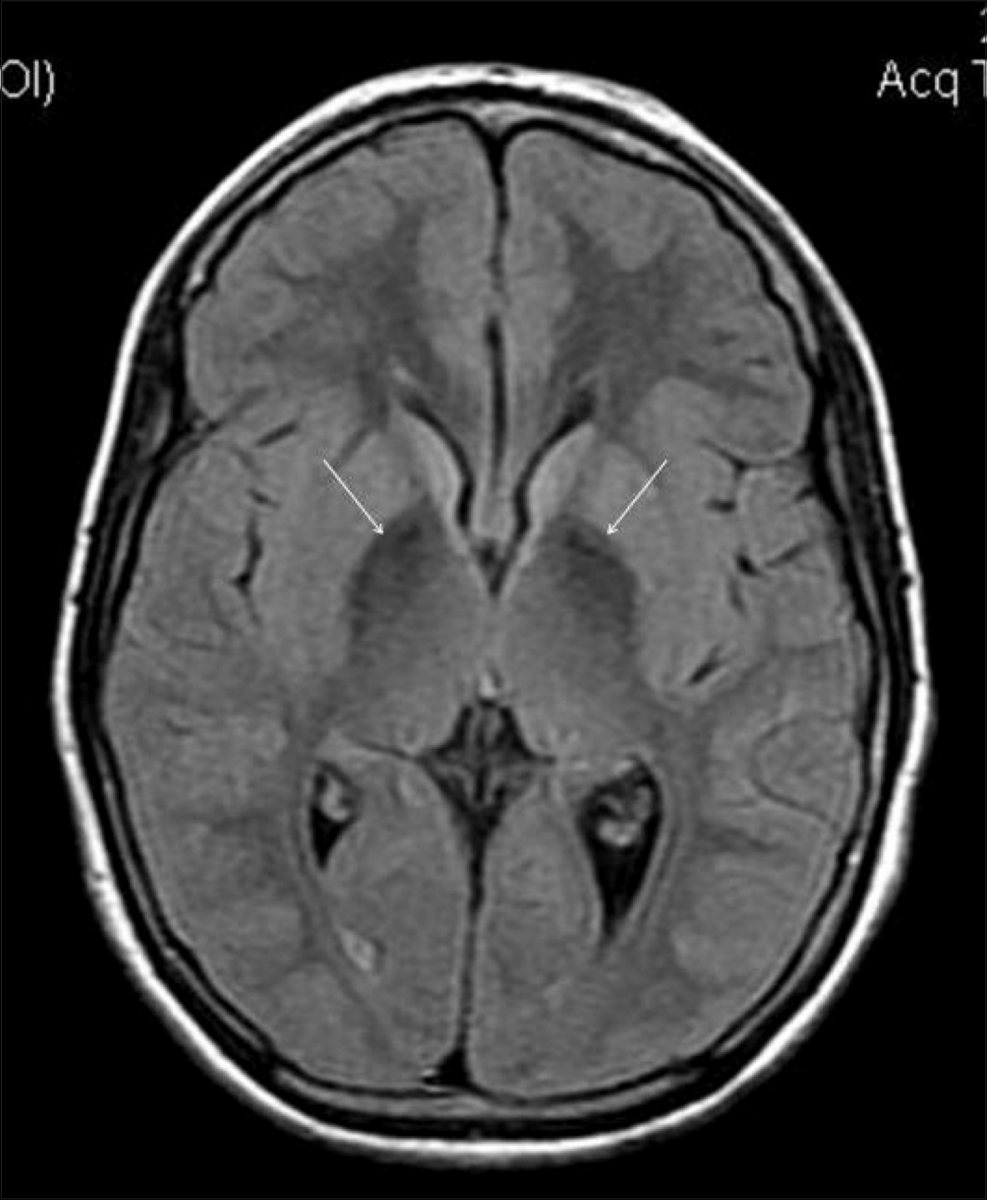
Axial FLAIR MRI image shows hypointense signals (arrows) within both globus pallidi
